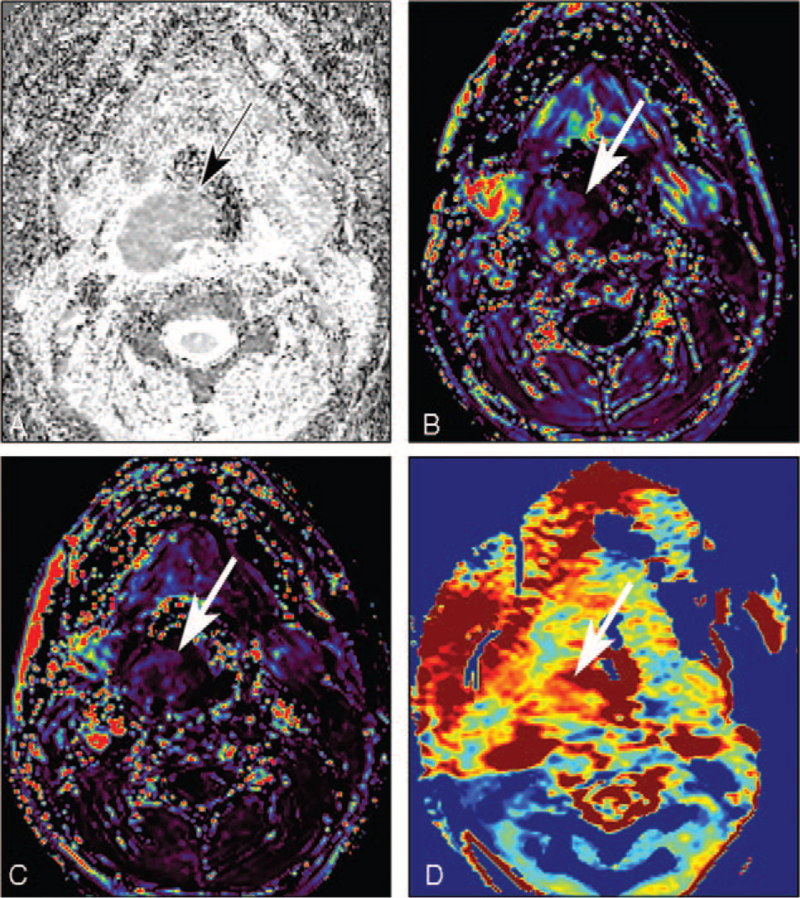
Advanced magnetic resonance imaging (MRI) findings. (A) The apparent diffusion coefficient (ADC) map derived from diffusion-weighted images shows a relatively low ADC value (0.76 × 10^−3^ mm^2^/s), indicating hypercellularity. (B, C) Maps derived from dynamic contrast-enhanced magnetic resonance images (MRI) show relatively low values of the volume transfer constant between the extracellular extravascular space and blood plasma (Ktrans, 0.04/min) and blood plasma volume per unit tissue volume (Vp, 0.004), which are characteristic of hypovascular tumors. (D) Amide proton transfer-weighted-MRI show a high amide proton transfer-weighted-signal of approximately 2.6%, suggesting a high protein/peptide content. The black arrow (A) and white arrows (B, C, D) indicate the mass.
